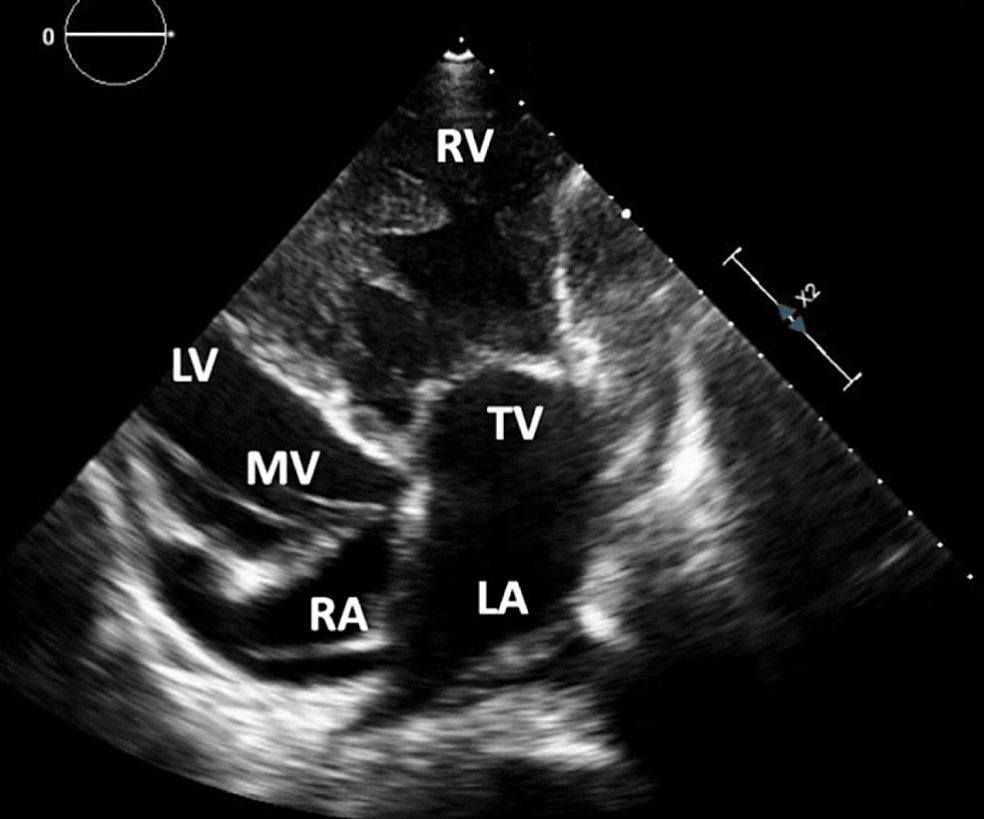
Echocardiography (ECHO) Evaluation of Cardiac Structure and Function in Congenitally Corrected Transposition of the Great Arteries (CCTGA) Congenitally corrected transposition of the great arteries featuring the tricuspid valve situated more apically on the right, which interfaces with a hypertrophied right ventricle.
